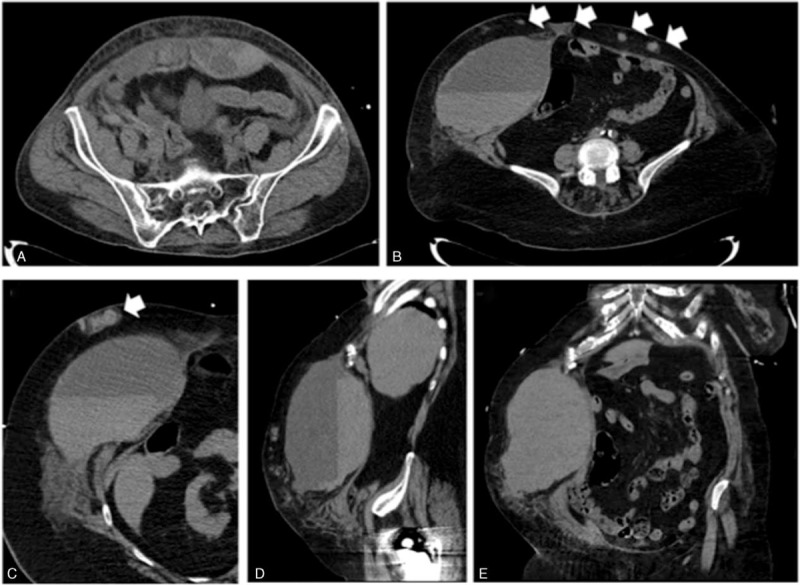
Computed tomography (CT)-scan imaging of bleeding in the anterior abdominal wall. (A) Bleeding in the rectus abdominis muscle. (B, C) Horizontal sections showing an important bleeding in the right rectus abdominis muscle associated with several subcutaneous hematomas (white arrows), sagittal section (D), and coronal section (E).
